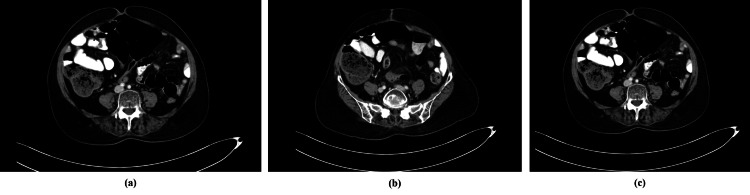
Contrast CT scan showed no pelvic mass or peritoneal nodules and no ascites.
